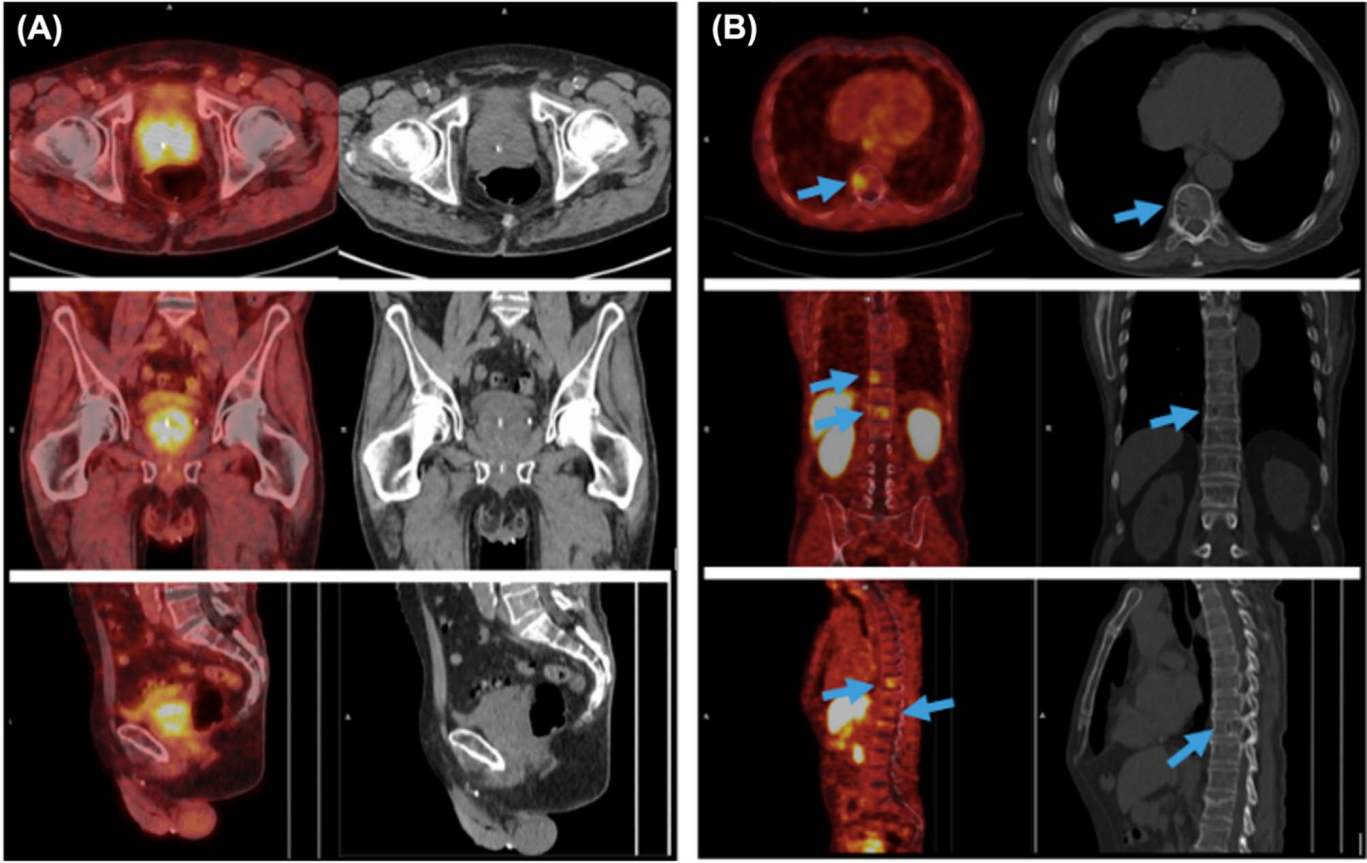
Primary staging of a treatment-naïve 75-year-old PCa patient with bone metastases, imaged using [^64^Cu]Cu-DOTAGA-PSMA PET/CT. At the time of imaging, PSA was 47 µg/L. (A) PSMA-positive primary tumor (SUV_max apical: 9.5; left base: 8.0). (B) PSMA-avid bone metastases in T10 and T12 (SUV_max: 5.3 and 4.6, respectively); arrow in low-dose CT image indicates the lesion in T10. Reproduced from Mirzaei et al. [103], licensed under CC BY 4.0 (https://creativecommons.org/licenses/by/4.0/)
